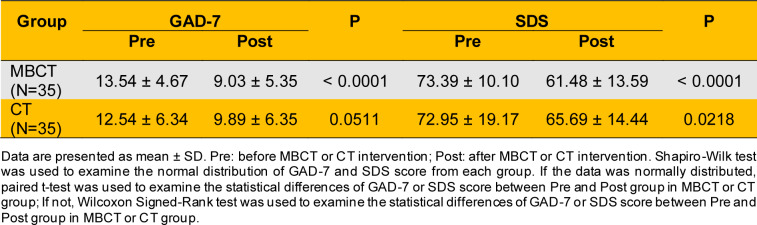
GAD-7 and SDS score of all patients with depression received MBCT or CT
